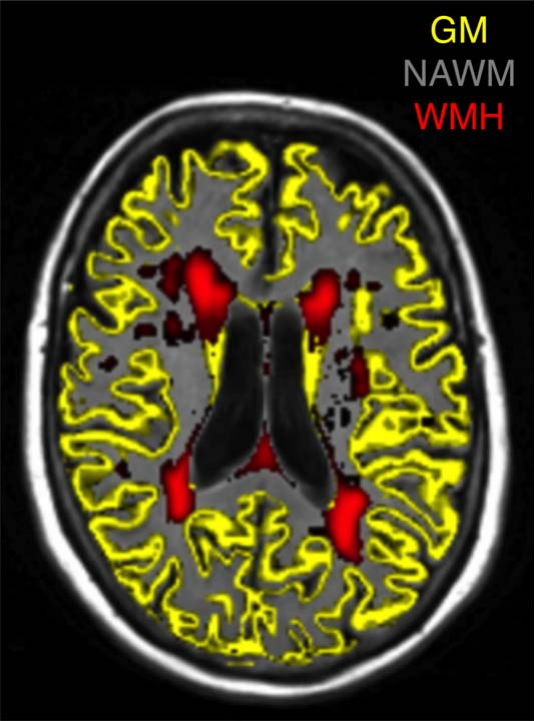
Use of multisequence (T1‐weighted and FLAIR‐weighted) brain magnetic resonance imaging (MRI) to produce tissue‐segmented images in one randomly‐selected participant from the present study. GM, grey matter; NAWM, normal‐appearing white matter; WMH, white matter hyperintensities.
